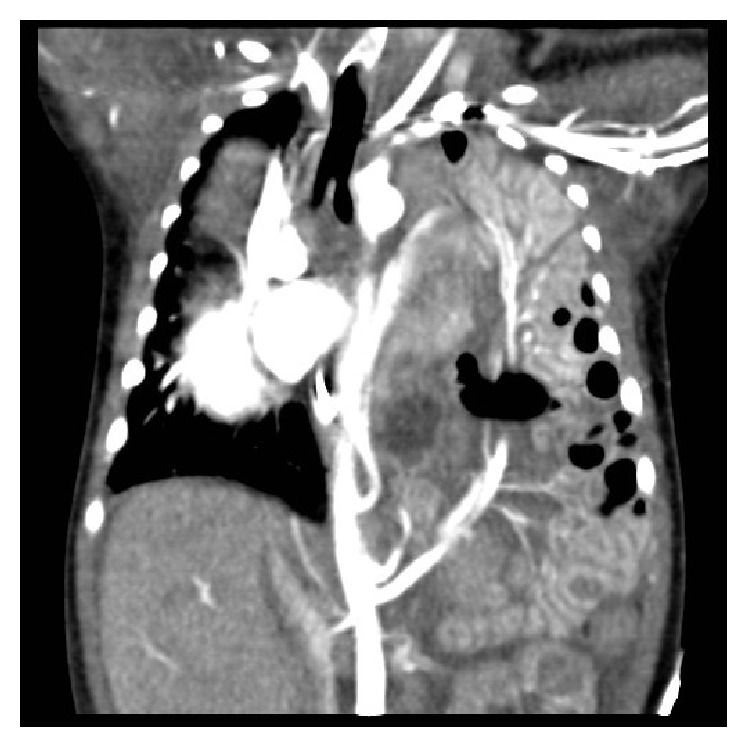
Coronal postcontrast CT image showing bronchopulmonary sequestration in a left paraspinal location. Herniated bowel loops and the superior mesenteric artery are seen in the left hemithorax. A branch from coeliac artery is seen supplying spleen, which is located in the left lower paraspinal region.
